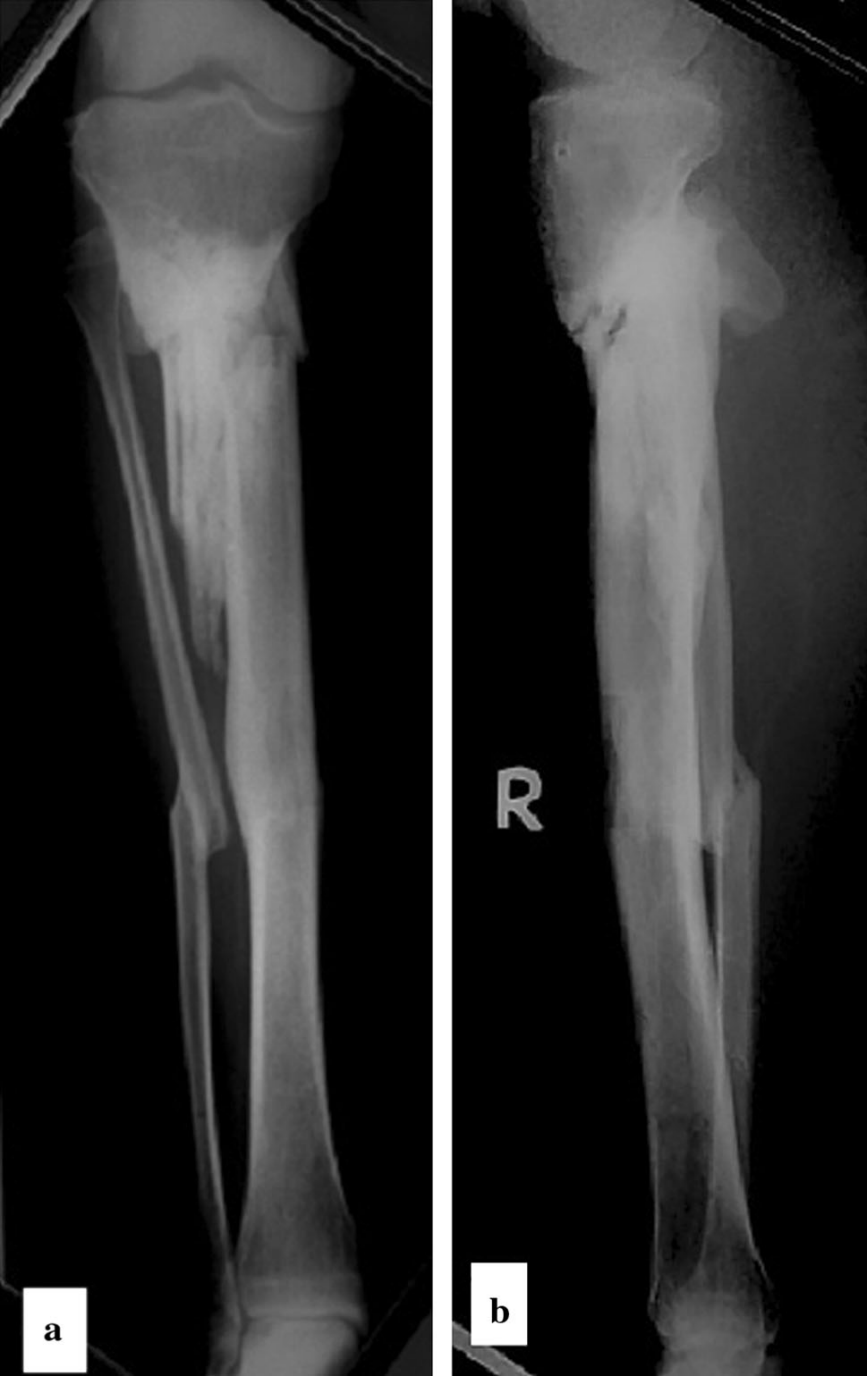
a, b Antero-posterior and lateral radiographs at final follow-up showing fully consolidated fracture and distraction sites with 10 mm medial translation of the mechanical axis
